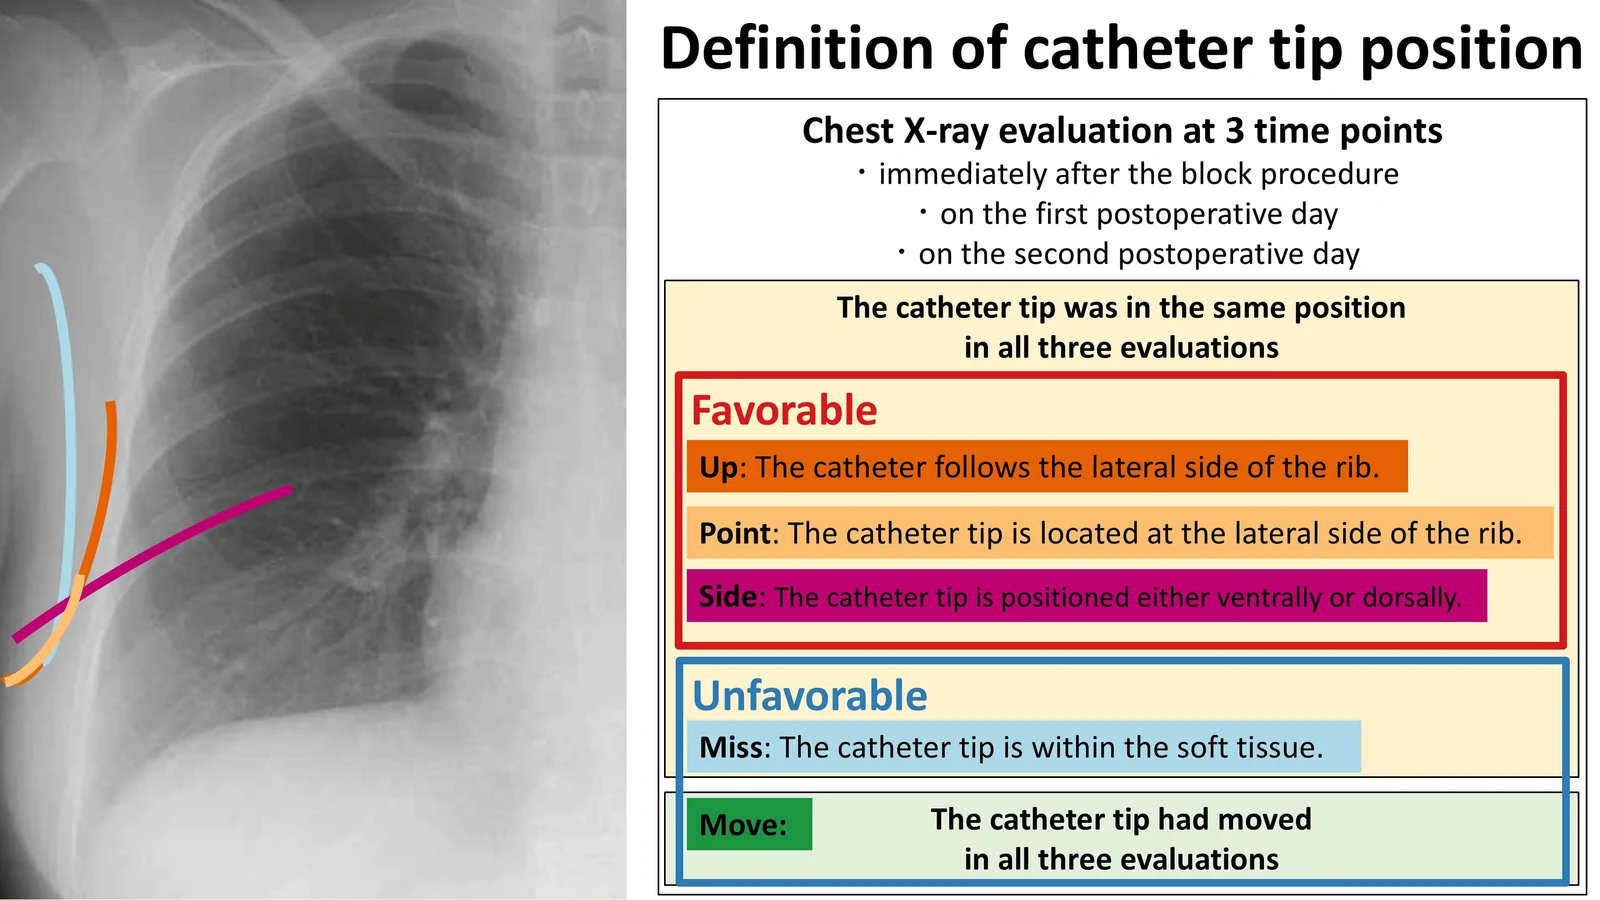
Evaluation of radiographic catheter alignment on chest radiographs Radiographic catheter alignment was assessed on serial chest radiographs obtained immediately after block placement and on postoperative days 1 and 2. “Up,” “Point,” and “Side” were classified as favorable alignment, whereas “Miss” and “Move” were classified as unfavorable alignment. This figure was manually created by the authors using Microsoft PowerPoint.
